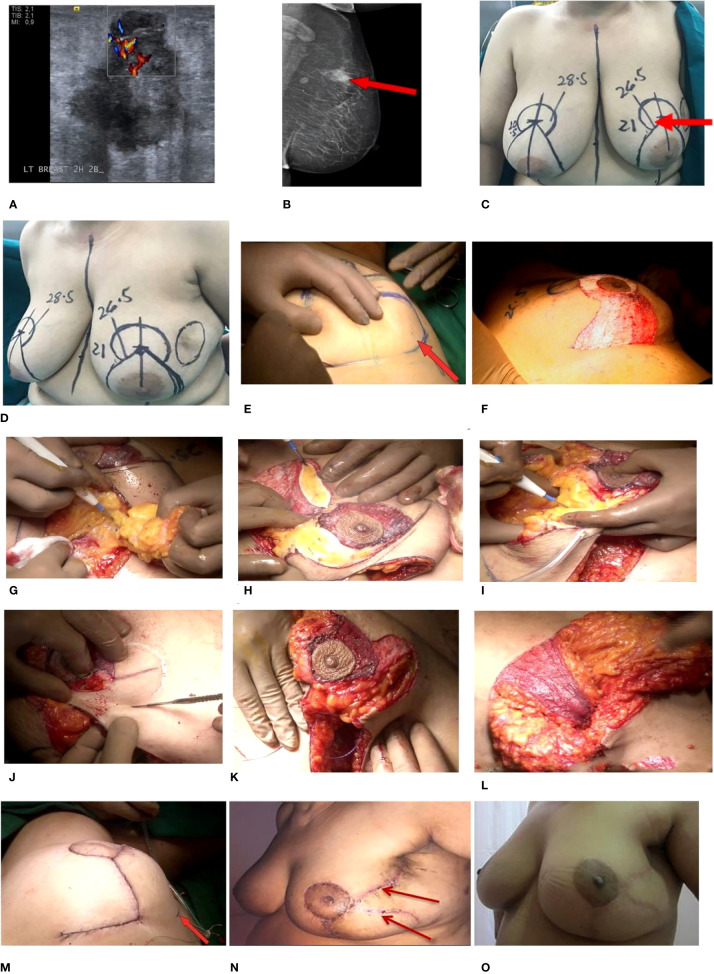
Split reduction TM. (A, B) Pre-operative radiology images. (A) 2D mammogram—MLO view of the left breast. Oval iso- to hyperdense lesion is seen in the upper quadrant of the left breast, which shows spiculations and a small enlarged left axillary node. (B) USG—a hypoechoic solid lesion, taller than wide is seen at the 2 o’clock 2B position of the left breast. (C, D) Markings also showing tumor in the UOQ with involved skin (arrow). (E–M) Intra-operative images. (E) No incision on lower outer part of IMF. (F) De-epithelialized inferior pedicle along with the medial wing. (G) Excision of the tumor along skin. (H) Dual pedicles being defined. (I) Delineating the superomedial pedicle. (J) Marking of the future NAC. (K) Connecting the tumor cavity and the lateral wing to create a continuum of skin. (L) Inferior pedicle being used as a filler. (M) Restructured breast, with the dermal incision in the lateral aspect taken inadvertently. Post-operative images at (N) 1-month follow-up and (O) 3-year follow-up. TM, therapeutic mammoplasty; MLO, mediolateral oblique; USG, ultrasonography; UOQ, upper outer quadrant; NAC, nipple–areolar complex.
